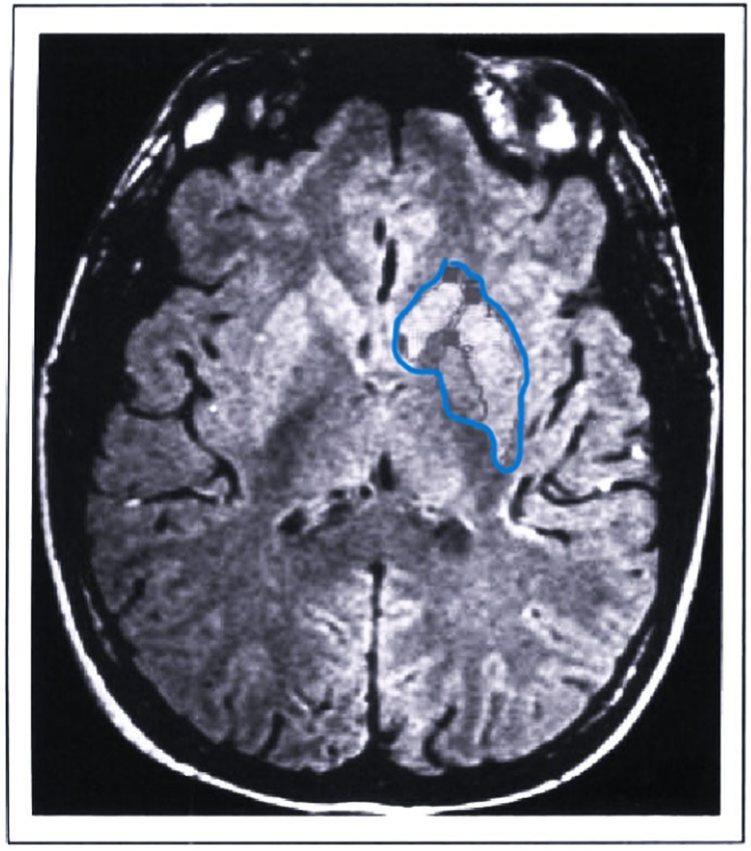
Two‐dimensional axial MR image, with spatial identification of the basal ganglia, circumscribed with the blue contour lineo.^19^ [Color figure can be viewed at wileyonlinelibrary.com]
